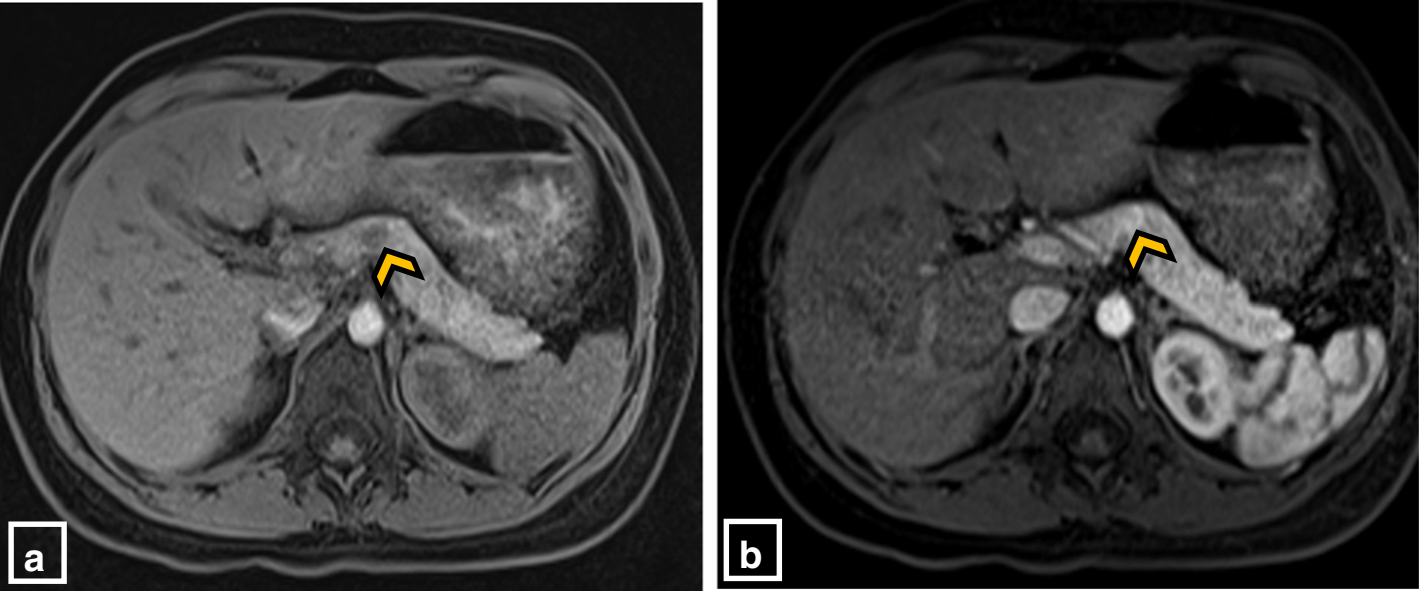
Result of MRI of the abdomen. Axial T1 weighted pre-contrast (a) and post-contrast (b) MRI images demonstrate a round enhancing lesion in the body of the pancreas (arrowhead)
